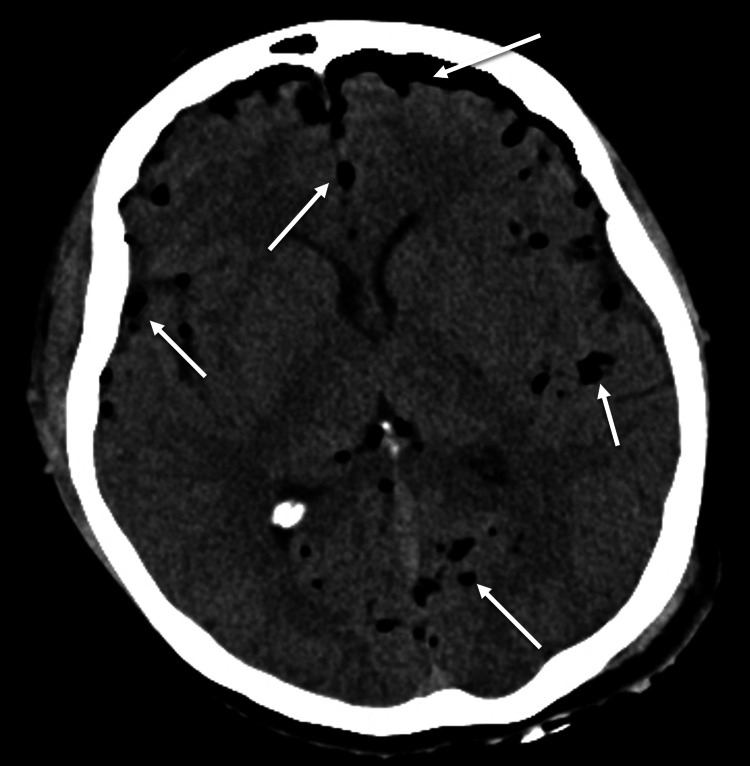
Initial head computed tomography showing pneumocephalus (arrows) without mass effect.
